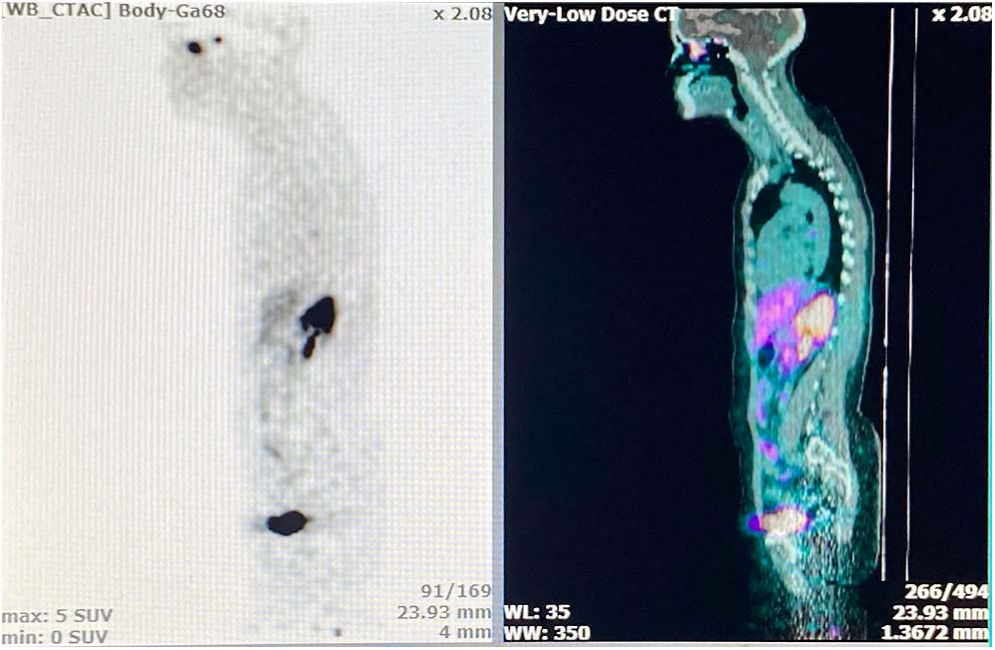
^68^Ga-DOTANOC PET-CT images showing intense hyperfixation projecting to the left ethmoidal cells.
